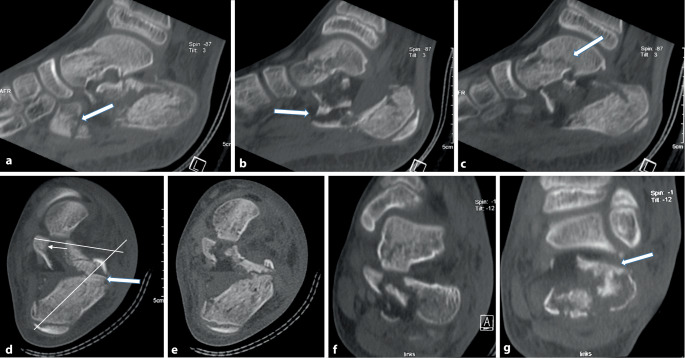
CT-Analyse:* komplexes Fußtrauma mit mäßig dislozierter Kuboidfraktur (a, *weißer Pfeil*), mehrfragmentärer Fraktur des Processus anterior calcanei mit Dislokation der Gelenkfläche zum Kuboid (b, *weißer Pfeil*), minimal dislozierter Talushals/Taluscorpus-Fraktur (c, *weißer Pfeil*), um 50° varisch disloziertem Tuber calcanei sowie Fraktur der medialen (d–f) und posterioren (g) Gelenkfacette des Subtalargelenks
